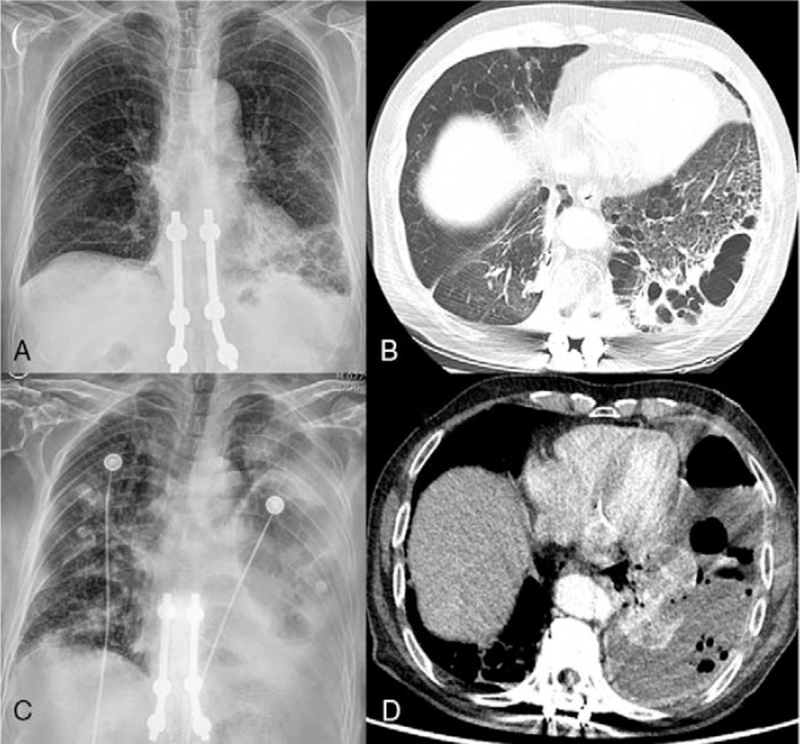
Chest radiograph and computed tomography (CT) images of case 1. (A) and (B) Images obtained 4 months before presentation. (C) and (D) Images obtained at presentation to the emergency room. (A) Chest radiograph showing consolidations in the left lower lobe. (B) Chest CT image showing a subpleural cavitary lesion with perilesional consolidation in the left lower lobe. (C) Chest radiograph showing a pleural effusion with air-fluid levels in the left hemithorax and consolidations in the right lung. (D) Chest CT images revealing a large pleural effusion with smooth wall thickening and enhancement suggestive of pleural empyema in the left lung.
